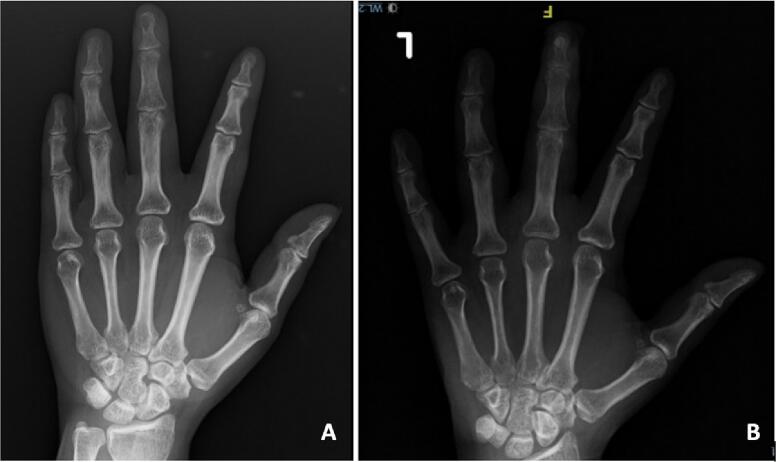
The anteroposterior radiograph shows bone destruction and cortical thinning in the distal phalanx of the left middle finger (A); the postoperative X-ray shows a synthetic bone substitute (B).
